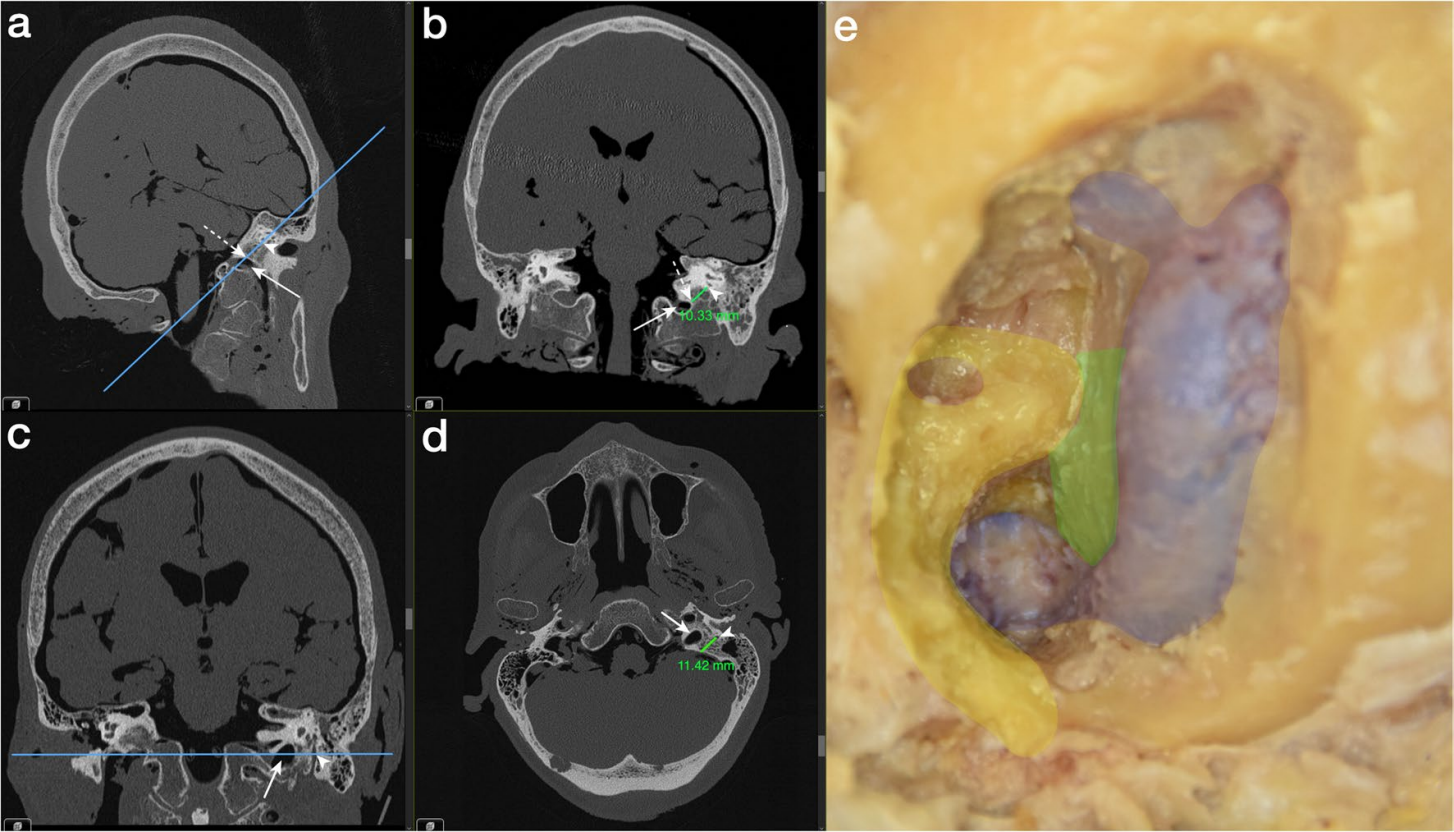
Annotated radiological measurements before dissection and anatomical overview of the infralabyrinthine access window on the left side a Reformatted and tilted sagittal and b cranial high-resolution computed tomography (CT) scans transecting the lower edge of the bony posterior semicircular canal (white arrowhead), the jugular bulb (white arrow), and the apex of the jugular bulb (dotted white arrow) are highlighted. The cutline (blue line) on the tilted sagittal scan overlies the minimal infralabyrinthine distance between the bony posterior semicircular canal and the apex of the jugular bulb, denoting the plane of the coronal image. The measured vertical distance (10.33 mm) is demonstrated on the coronal image. c Reformatted and tilted coronal and d axial cranial high-resolution CT scans transecting the jugular bulb (white arrow) and the midpoint of the fallopian canal (white arrowhead) are displayed. The cutline (blue line) on the tilted coronal scan, intersecting the midpoint of the fallopian canal, indicates the axial cut plane. The measured horizontal distance (10.33 mm) running parallel to the trajectory of the jugular bulb and extending between the midpoint of the fallopian canal and the presigmoidal dura is shown on the axial image. e Color marked overview of the infralabyrinthine access window with preserved osseous posterior semicircular canal and fallopian canal (yellow), sigmoid sinus and bone-covered jugular bulb (blue), and presigmoidal dura (green)
